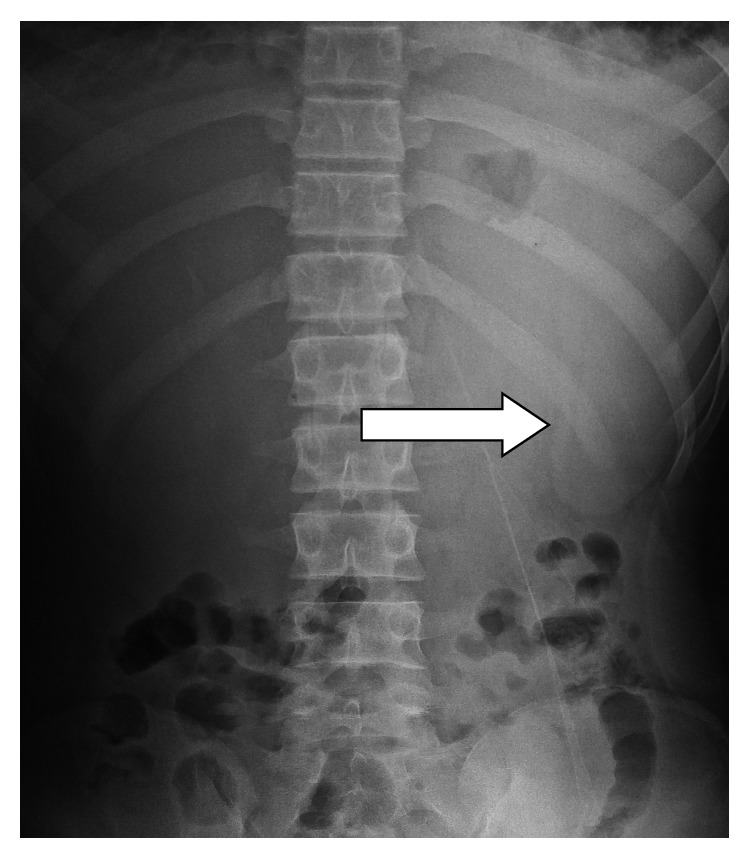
Plain abdominal radiograph with obvious outline of spleen associated with AP and AH.
